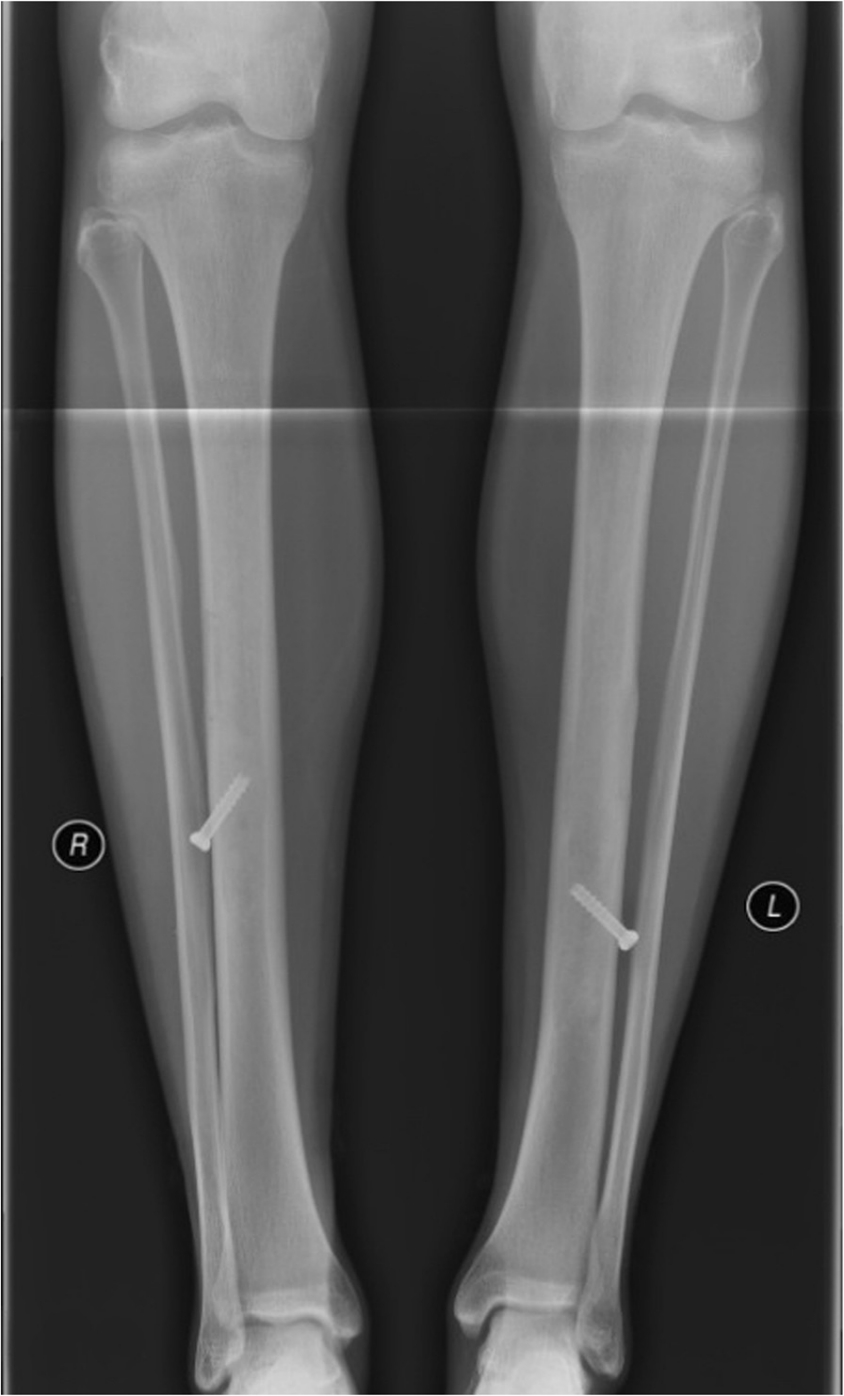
Follow-up x-rays a.p. 6 month (left leg) and 3 month (right leg) postop
